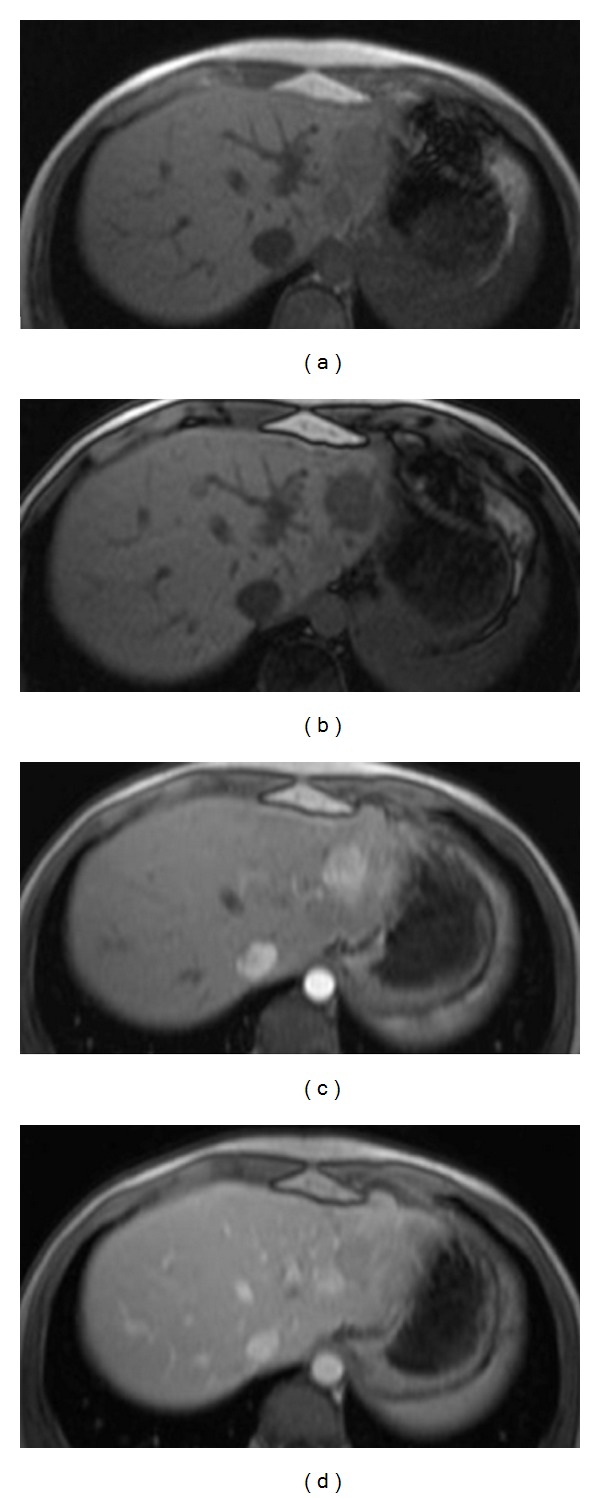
Adenomatosis related to HNF1-*α* germline mutation aspect on MRI in a 14-year-old girl: (a) and (b): T1 WI with chemical shift shows two lesions in the left lobe of the liver with drop of the signal of the largest lesion on the out-phase sequence that reveals the presence of fat in the tumor. (c) and (d): T1 WI after contrast injection shows early arterial enhancement of the largest lesion with washout in the late portal phase.
